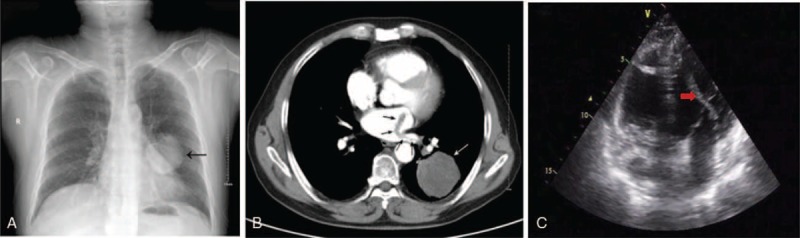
A chest radiograph (A) and computed tomography scan (B) showed a mass of 5.5 cm in the left lower lobe and a linear band of 2.0 cm behind the LA (arrows). Transthoracic echocardiography (C) displayed 8 to 10 cm embolus extension (red arrow), highly mobile, from the LA prolapsing to the left ventricle. LA = left atrium.
